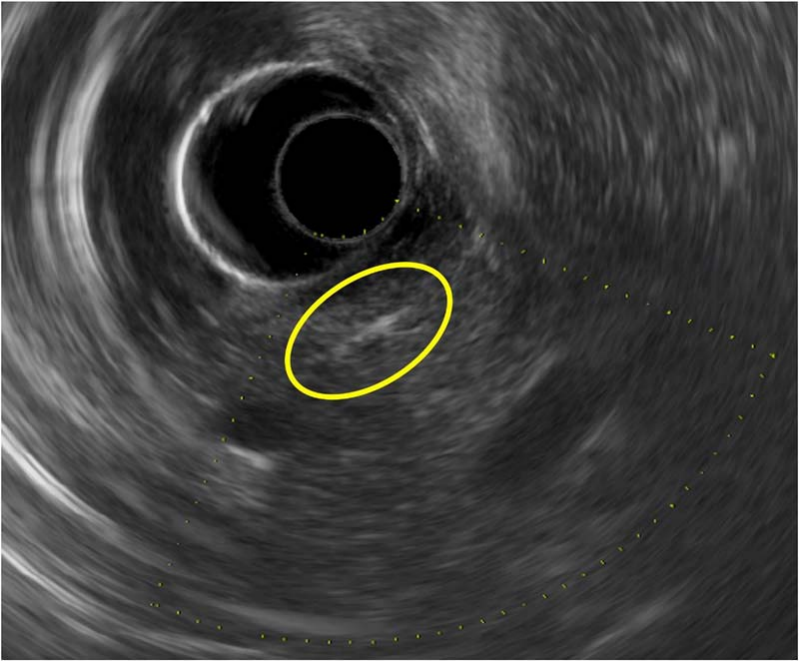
A linear and straight highly echogenic substance is seen in the parenchyma of the pancreatic head on endoscopic ultrasonography.
